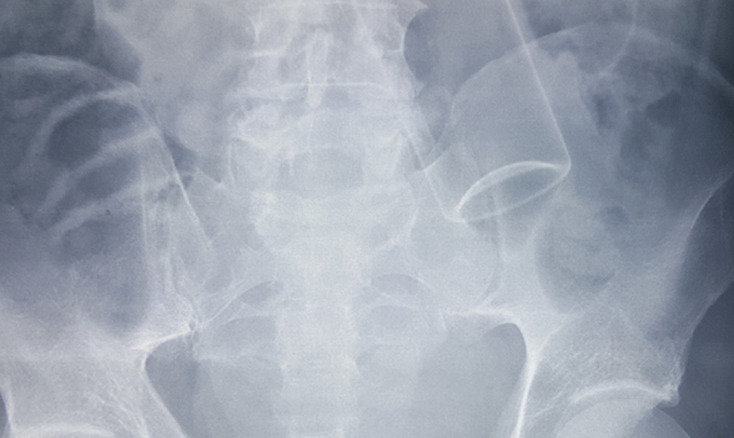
pelvic X-ray showing a left colon foreign body
